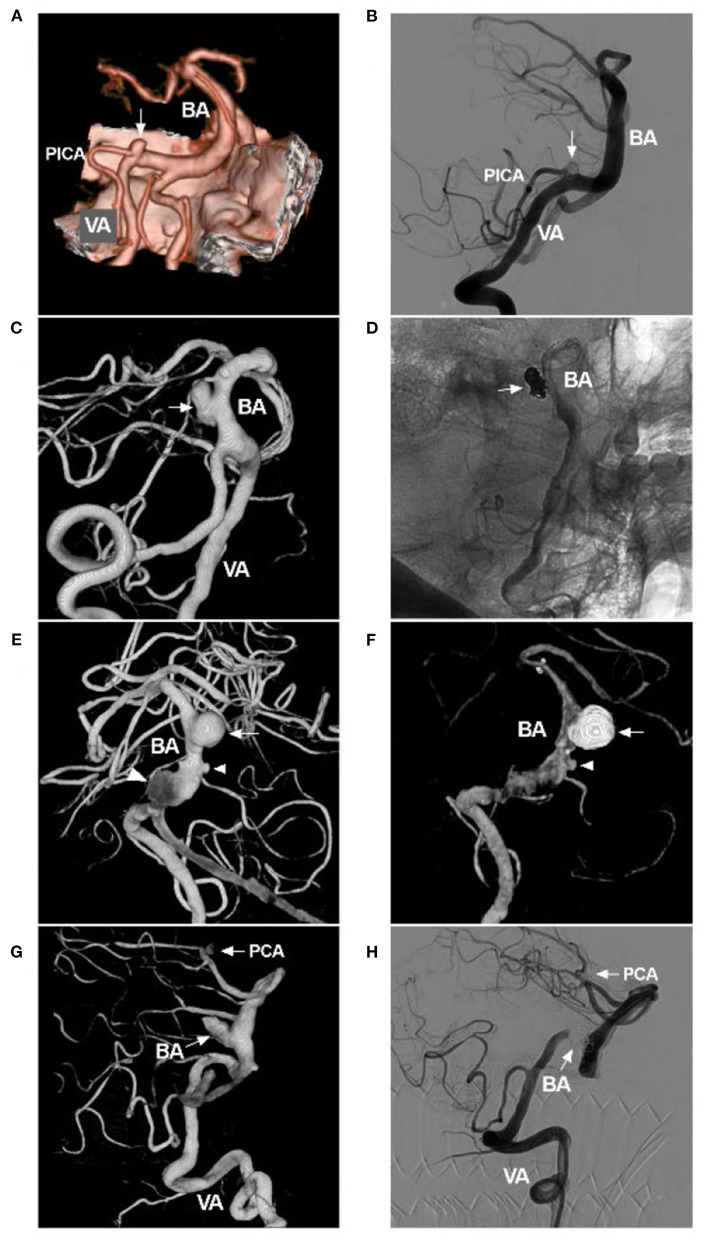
Coiling saccular aneurysms in VBD. (A) Pre-operative CTA showing an aneurysm (arrow) of PICA origin in VBD. (B) Post-operative DSA showing that the aneurysm (arrow) was coiled with stenting assistance. (C) Pre-operative three-dimensional DSA showing an aneurysm (arrow) of the BA in VBD. (D) Post-operative DSA showing that the aneurysm (arrow) was coiled with stenting assistance. (E) Pre-operative three-dimensional DSA showing multiple tandem aneurysms, a large saccular aneurysm (arrow), a small aneurysm (small arrowhead), and a fusiform aneurysm (large arrowhead). (F) Post-operative half-year follow-up DSA showing acceptable embolization with stenting assistance of the large saccular aneurysm (arrow); the small aneurysm did not increase in size. (G) Pre-operative three-dimensional DSA showing two aneurysms (arrows with BA and PCA) of the BA and PCA. (H) Post-operative DSA showing that the two aneurysms (arrows with BA and PCA) were coiled, the one in the BA requiring stenting assistance. This VBD made treating the PCA aneurysm difficult because of the elongation and tortuosity of the access route. BA, basilar artery; CTA, computed tomography angiography; DSA, digital subtracted angiography; PCA, posterior cerebral artery; PICA, posterior inferior cerebellar artery; VA, vertebral artery; VBD, vertebrobasilar dolichoectasia.
